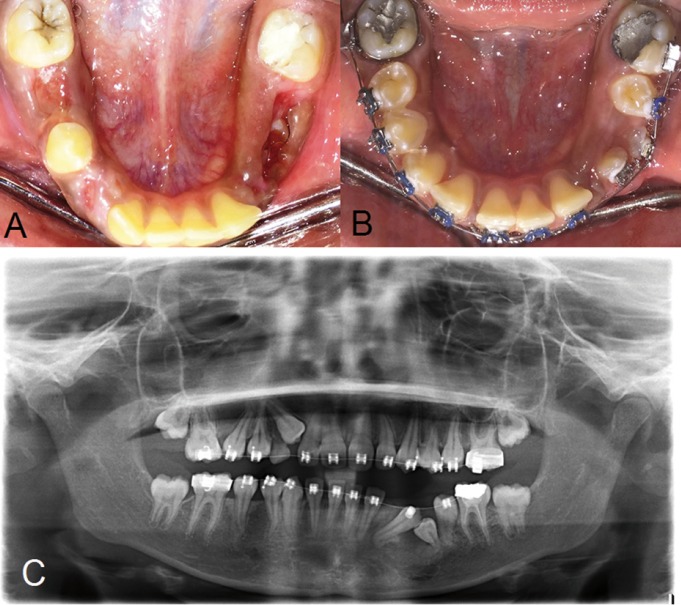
A) Soft tissue healing after removal of the pack of gauze in the 5th week post-operatively. B,C) Clinical picture and panoramic radiograph after five months of orthodontic treatment which revealed the up-righting of 35 and slight spontaneous eruption of 34.
